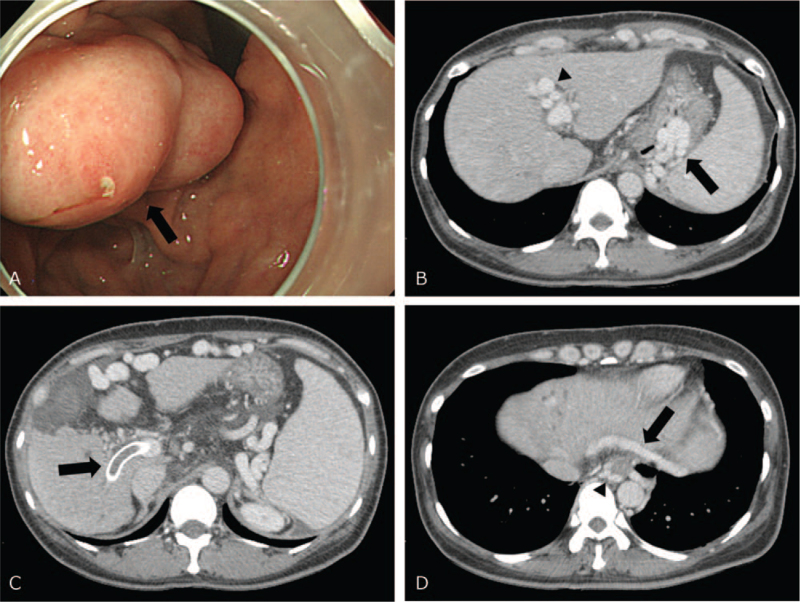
(A) Endoscopic image shows a large polypoid mass with stigmata (arrow) without active bleeding on the stomach. (B) CT shows underlying liver cirrhosis with splenomegaly, umbilical varix (arrowhead), and gastric varix (arrow). (C) A previously inserted TIPS stent (arrow) is occluded by a thrombus. (D) Only gastrocaval shunt (arrow) and esophageal varix (arrowhead) are seen.
